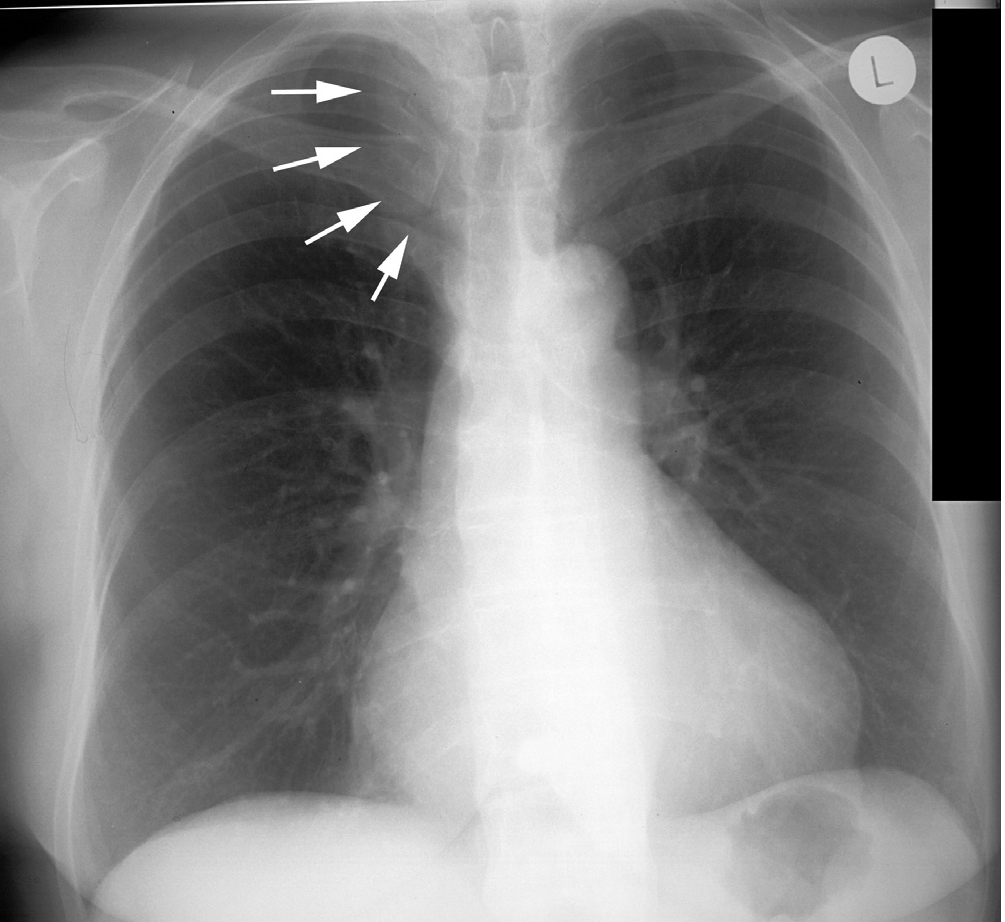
Preoperative chest x-ray displayed a mediastinal enlargement towards the right (→).
